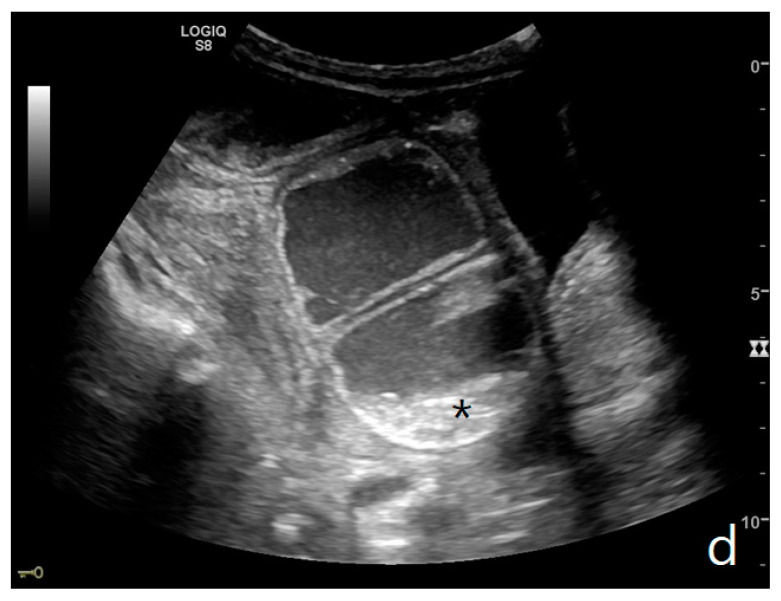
A complicated SBO in a 69-year-old male with gastric cancer and peritoneal carcinosis. Ultrasound images show long (a) and axial (b) evaluations of a fluid-filled, dilated small bowel loop with hyperechogenic floating material (shown with an asterisk) (b,d). Bowel peristalsis was absent. Mild parietal and valvulae conniventes thickening are present (c,d). Downstream loops present normal caliber (bowel jump diameter). Free fluid is interposed between bowel loops (black arrow) (b).
